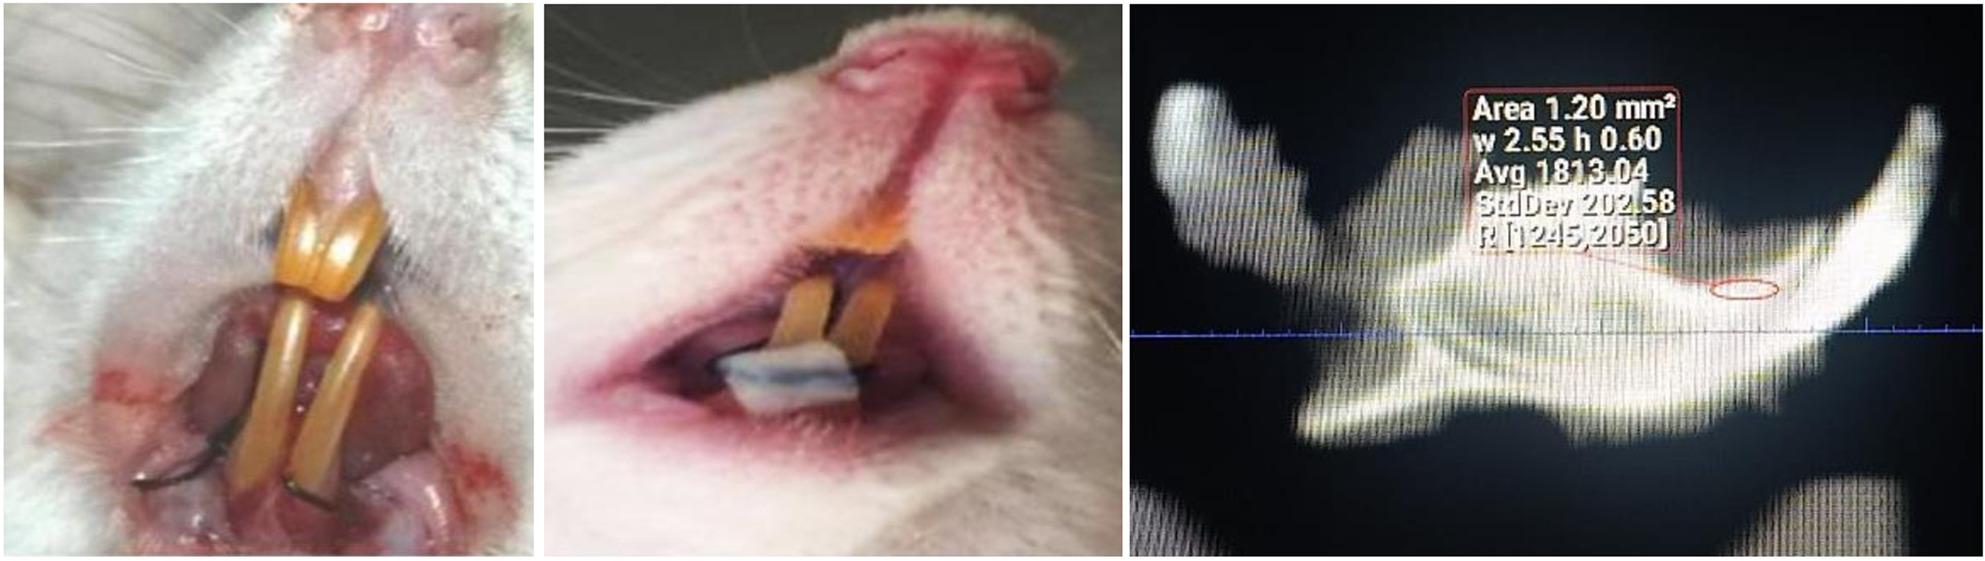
Clinical and radiographic images demonstrating an established periodontitis
